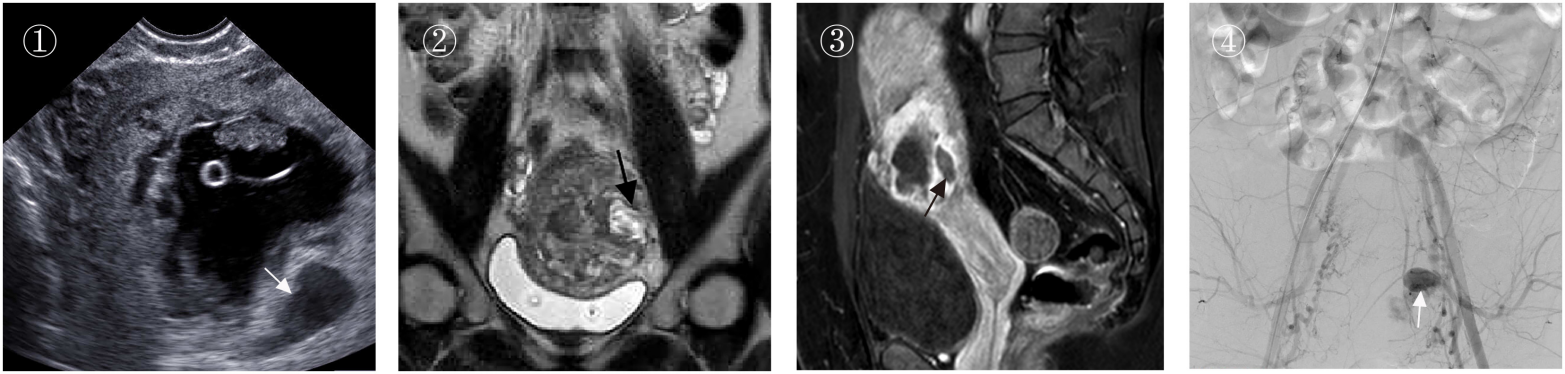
A case of type III cesarean scar pregnancy (CSP). (1). Preoperative color Doppler flow imaging ultrasound (CDFI-US) showed that the gestational sac presented a cystic-solid appearance, with an embryonic bud visible inside the sac, and a pseudoaneurysm (indicated by the white arrow); (2). Coronal T2-weighted imaging (T2WI) revealed the cystic-solid gestational sac, with multiple tortuous blood vessels around it, and a pseudoaneurysm (indicated by the black arrow); (3). Contrast-enhanced sagittal T1-weighted imaging (T1WI) showed cystic-solid enhancement within the gestational sac, with multiple tortuous blood vessels around the sac, as well as a pseudoaneurysm and its flow void effect (indicated by the black arrow); (4). Digital subtraction angiography (DSA) of the right uterine artery demonstrated the blood supply to the gestational sac, with visualization of the pseudoaneurysm (indicated by the white arrow) and the blood vessels around the sac.
